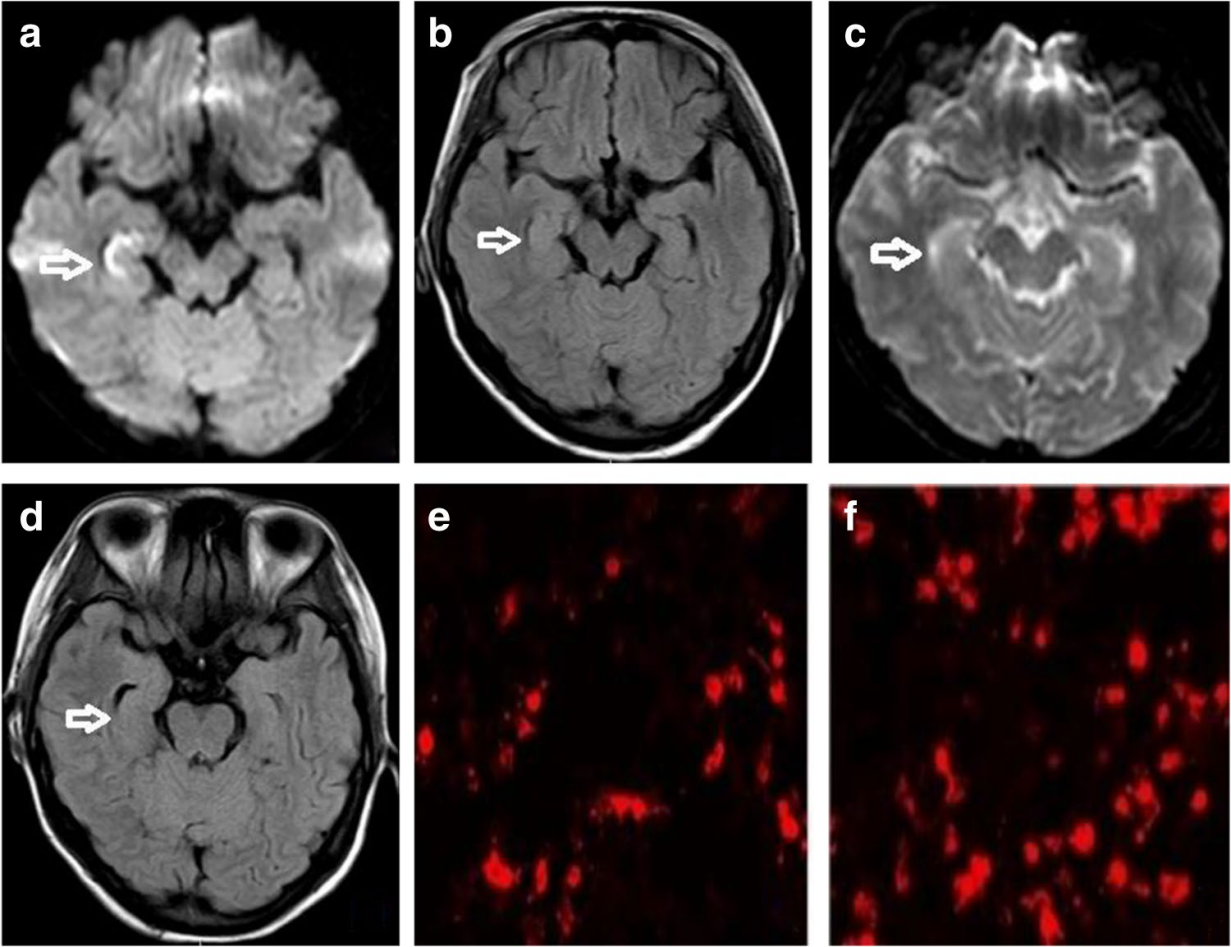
Symptoms and auxiliary examination in a patient with anti-NMDAR encephalitis. a Diffusion-weighted imaging of right hippocampal hyperintensity on the first MRI. b High signal intensity in right hippocampus of patients with FLAIR sequence on the first MRI. c The sequence of DWI in the second reexamination of MRI. d The sequence of FLAIR in the second reexamination of MRI. e The titer of anti-NMDAR antibody is 1:32. f The titer of anti-NMDAR antibody is 1:320
